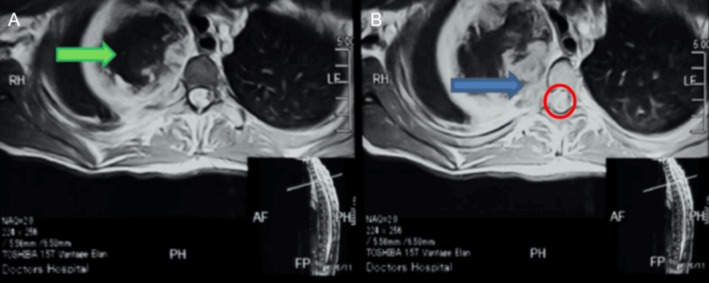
CE MRI showing (A) a large cystic mass with solid nodular peripheral enhancement (green arrow). (B) Spinal canal extension (blue arrow) with compression upon the cord and abnormal signals in the cord. The red circle shows abnormal signs in the cord.
